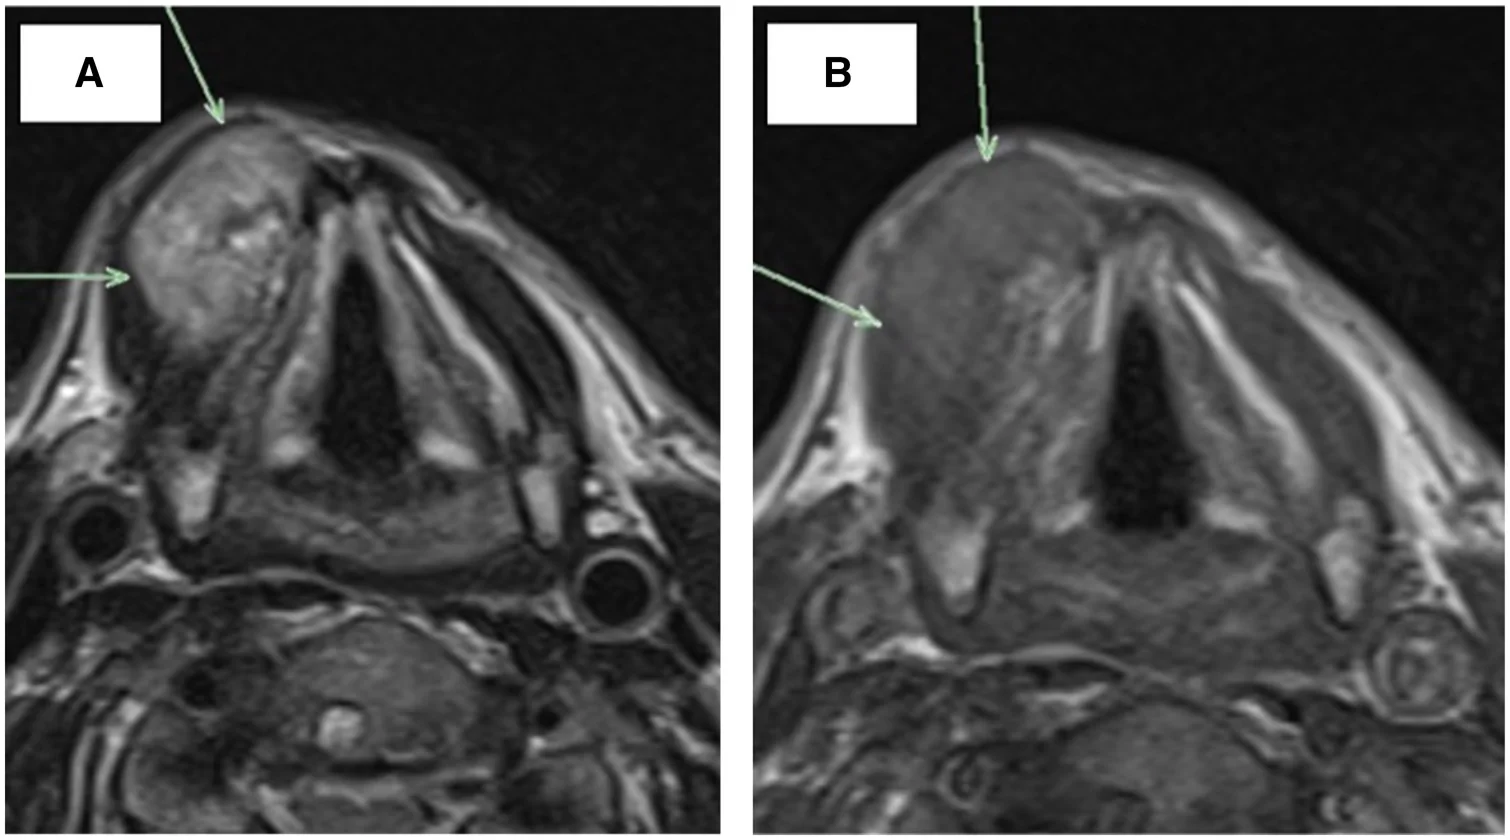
(A) Axial T2-weighted MRI of the neck. MRI demonstrates a 26 × 17 mm well-defined T2 hyperintense mass arising from the anterosuperior aspect of the right thyroid cartilage lamina. The lesion contains internal low-signal foci corresponding to calcifications and shows no extension into the paraglottic space or laryngeal vestibule. (B) Axial T1-weighted MRI of the neck. The lesion demonstrates intermediate signal intensity on T1-weighted imaging, with areas of internal higher signal intensity at its origin from the anterosuperior thyroid cartilage.
